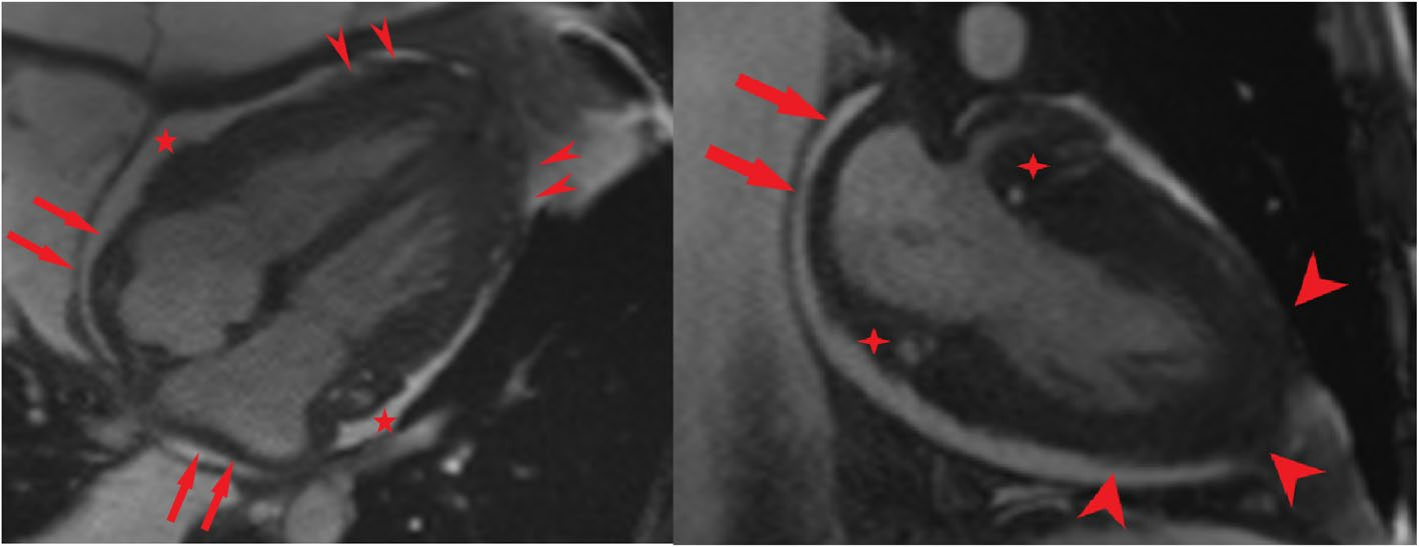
Cardiac magnetic resonance imaging shows moderate pericardial effusion and mild diffuse thickening of the parietal pericardium and infiltrative irregular enhancing thickening of the visceral pericardium (arrowhead). There are diffuse infiltrative thickening of the bi-atrial walls (arrow) and periarterial infiltration around the coronary arteries (asterisk)
